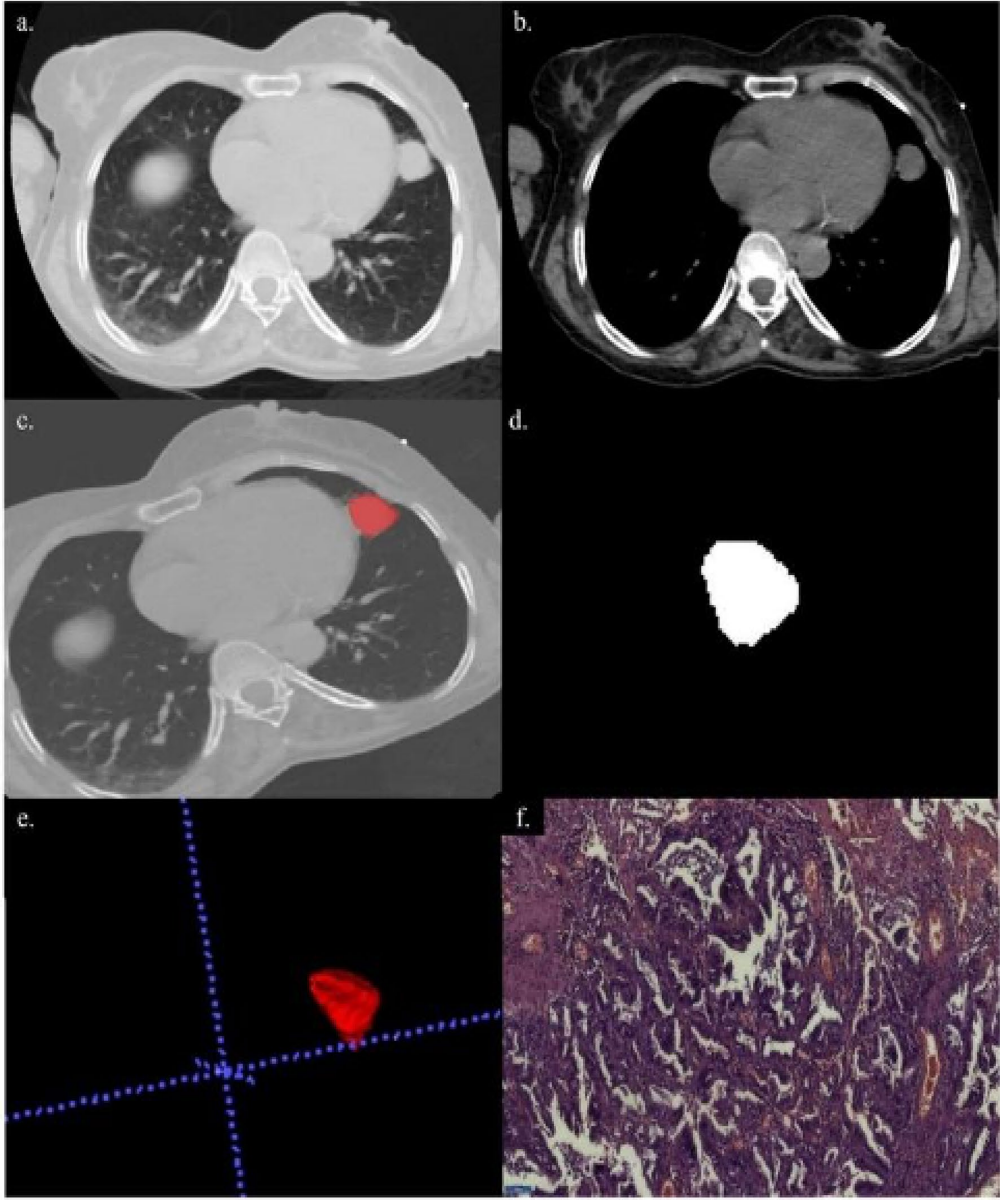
A 54-year-old female with complaints of cough. (a, b) Axial section of CT thorax in lung window and soft tissue window showing a nodule in the lingular segment of the upper lobe. (c) Creation of ROI for segmentation. (d) 2D-segmented nodule. (e) 3D-volumetric rendering of the nodule. (f) HPE: Adenocarcinoma lung.
